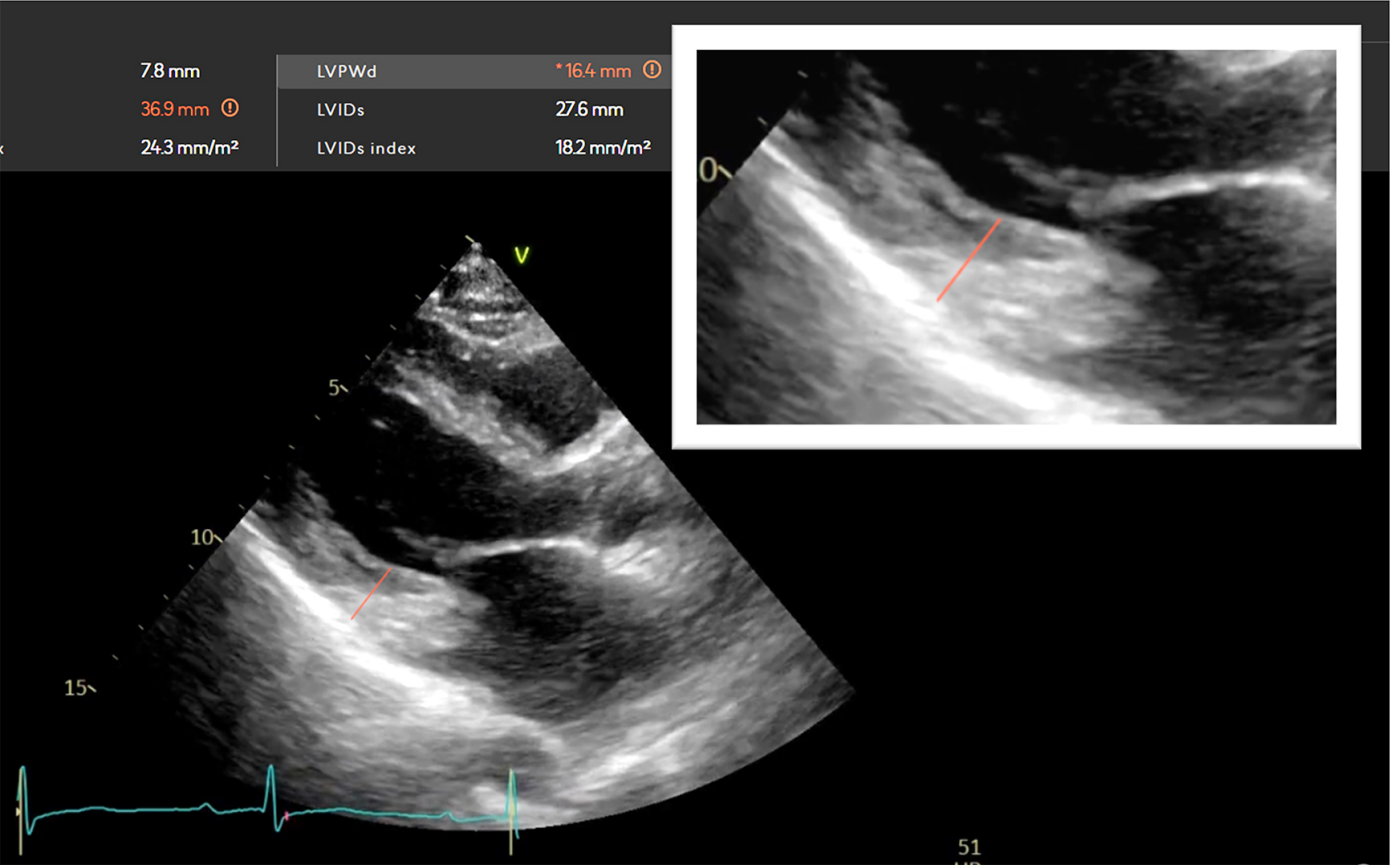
Erroneous automated measurement case of the posterior wall using AI Inclusion of chordae tendineae in the measurement results in a wall thickness overestimation (16.4 mm), potentially leading to a misdiagnosis of concentric hypertrophy. This case highlights the necessity for an accurate anatomical identification in AI-driven echocardiography.
